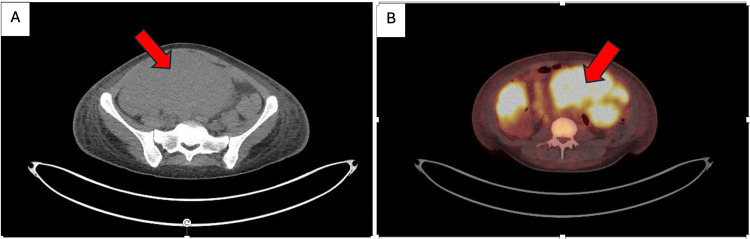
PET/CT Imaging demonstrating the hypermetabolic abdominal mass (A) Axial CT highlighting the lesion. (B) Fused axial PET/CT view confirming the hypermetabolic nature of the lesion
